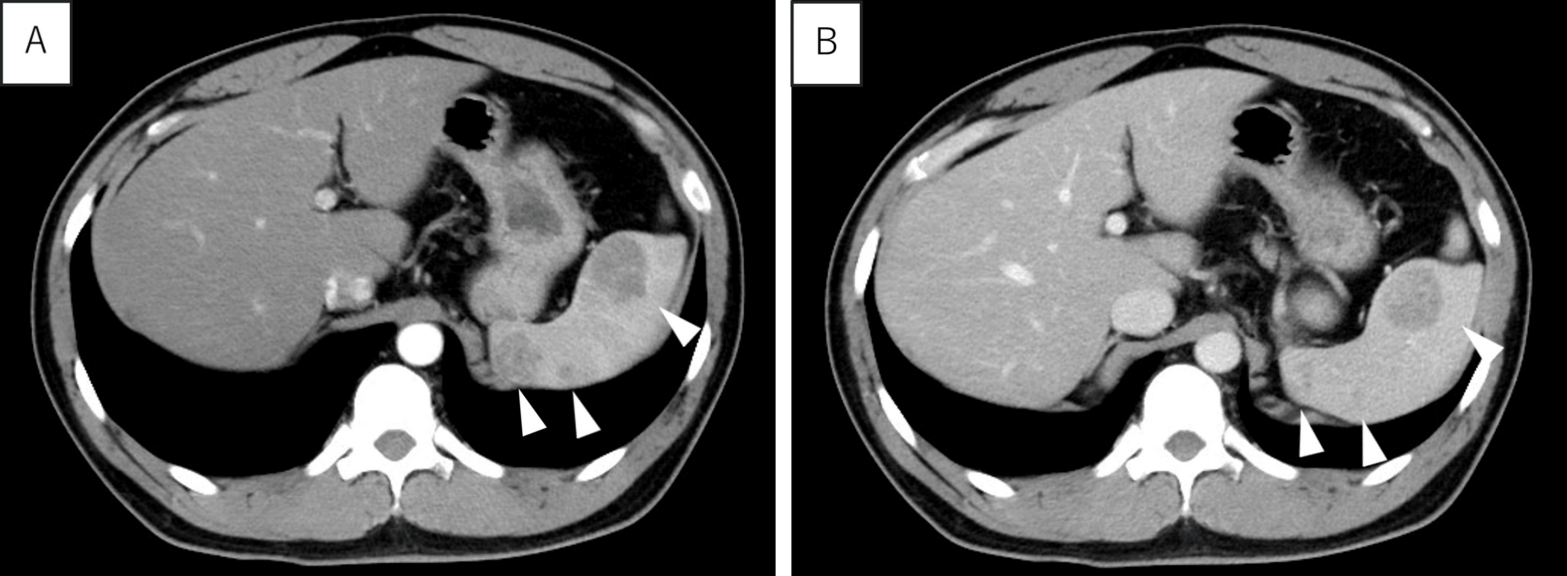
Contrast-enhanced CT CT showed three masses measuring 3.5 cm x 2.7 cm in the hilum, 2.3 cm x 2.1 cm in the upper pole, and 0.5 cm x 0.5 cm, with clear boundaries and poor contrast in the early phase (A) and late phase (B).
